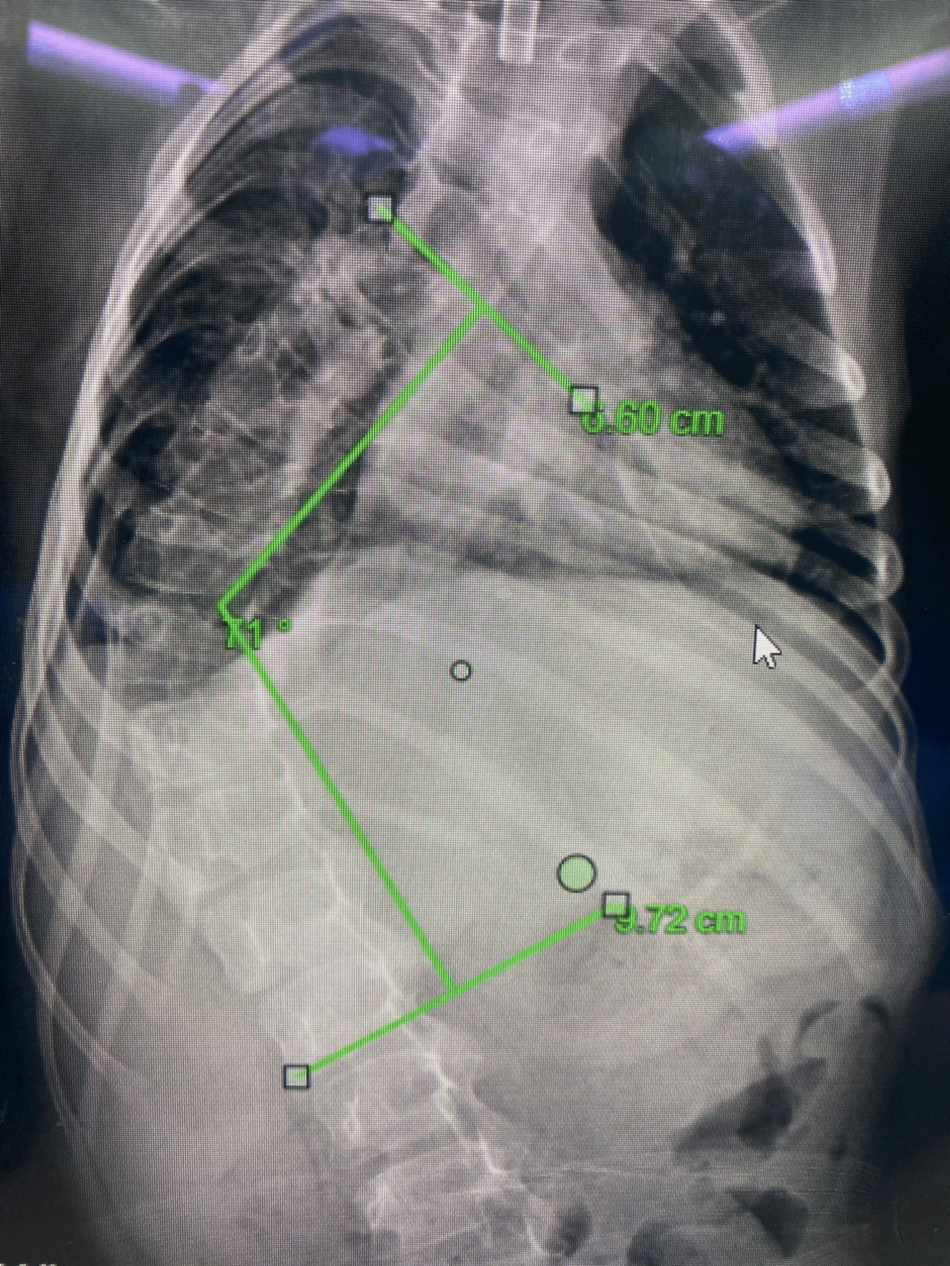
Preoperative AP thoracic and lumbar spine radiograph depicting the preoperative Cobb angle AP: Anteroposterior
